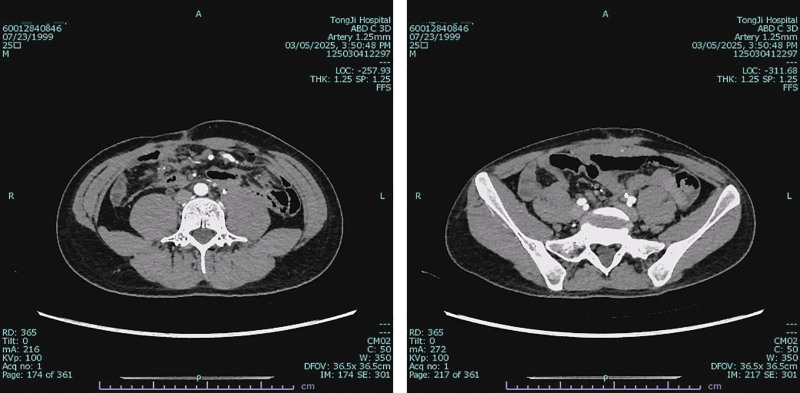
Case 2 patient’s follow-up CT image 3 years after surgery and initiation of systemic therapy. cDNA = complementary deoxyribonucleic acid, CT = computed tomography.
